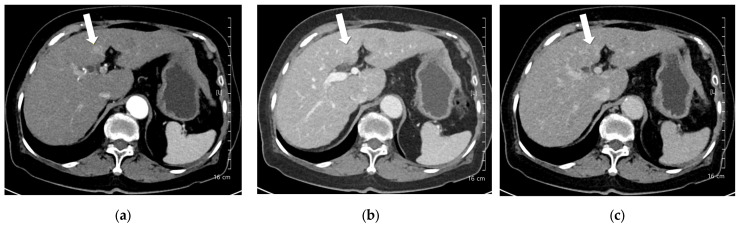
A 91-year-old woman with hepatocellular carcinoma (HCC). CT was performed using low-concentration iodine contrast material (total iodine dose, 518.4 mg I/kg). Overall image quality in the arterial and portal phases was acceptable. (a) An 8-mm enhancing nodule (arrow) is visible on the arterial phase at 100 kVp; (b,c) The nodule shows washout on the portal and delayed phases (arrows). Both reviewers classified the lesion as hepatocellular carcinoma.
